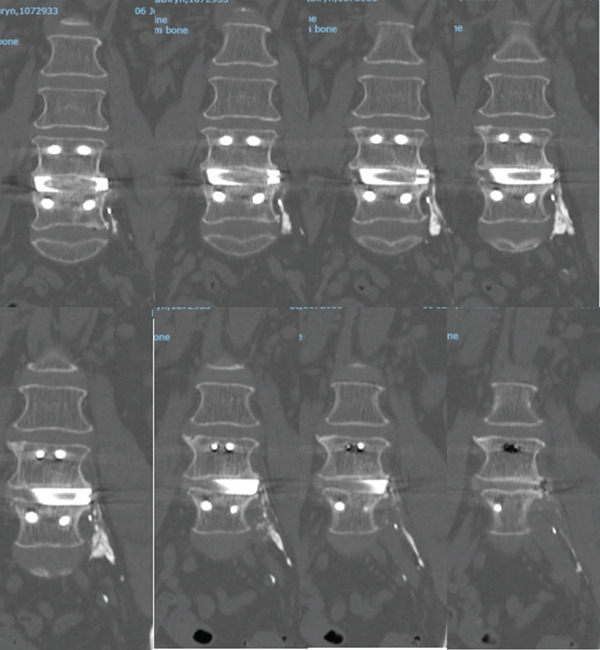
Coronal CT displaying extruded iFactor putty.
